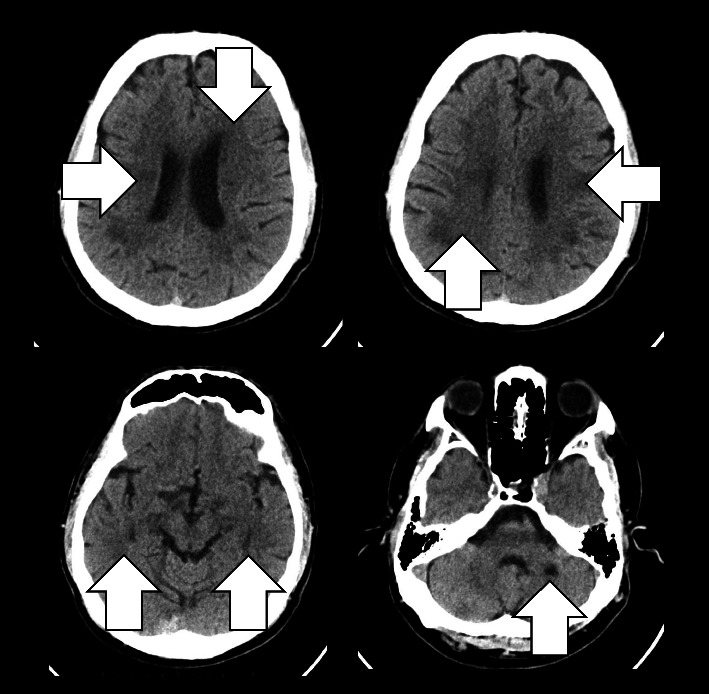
Solid arrows show multiple hypodense lesions of the white matter, which suggest an infarction on the CT brain.
